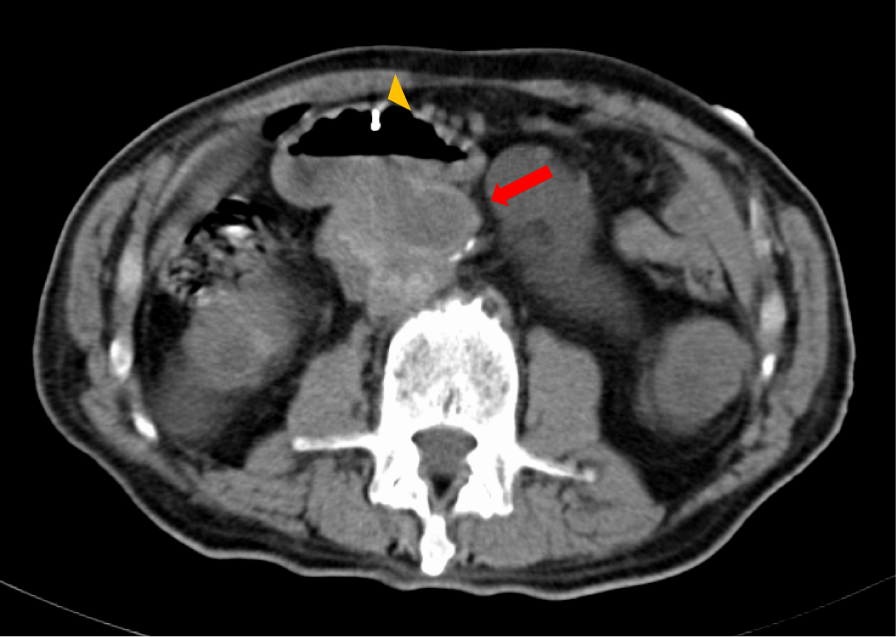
Simple computed tomography (CT) image after an upper endoscopy (case 6). Arrowhead: a cyst-shaped aneurysm adjacent to the duodenum is seen. Arrowhead: since a marking clip was placed on the opposite side, diagnosis of aorto-duodenal fistula was easy, even with a simple CT
